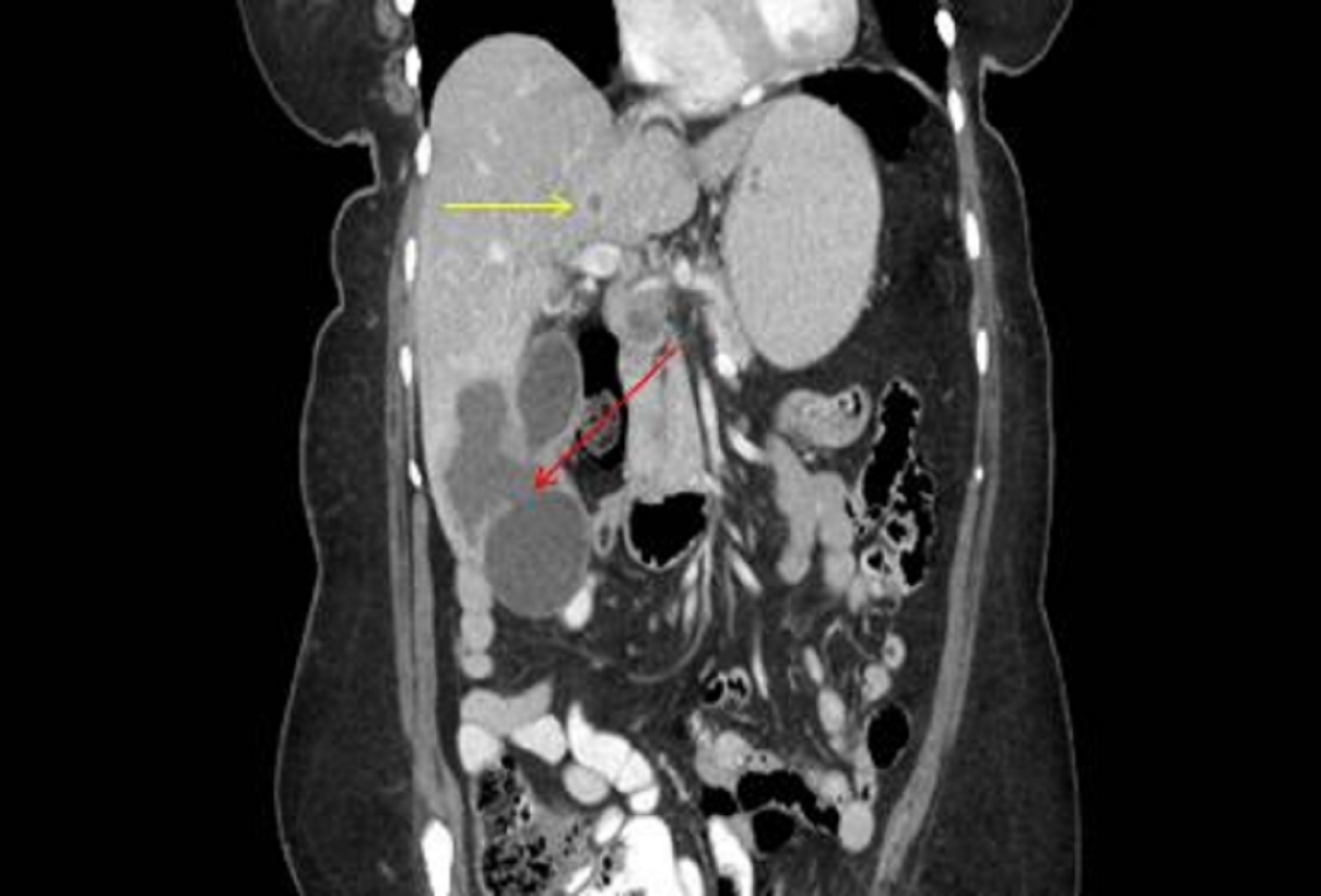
CT scan. The image demonstrates a coronal CT showing a hypodense mass with direct invasion into the right hepatic lobe (red arrow), with additional small lesion in the left hepatic lobe (yellow arrow). Not shown is portohepatous and peripancreatic lymphadenopathy. The extent of disease suggests an element of patient denial of symptoms prior to clinical presentation.
